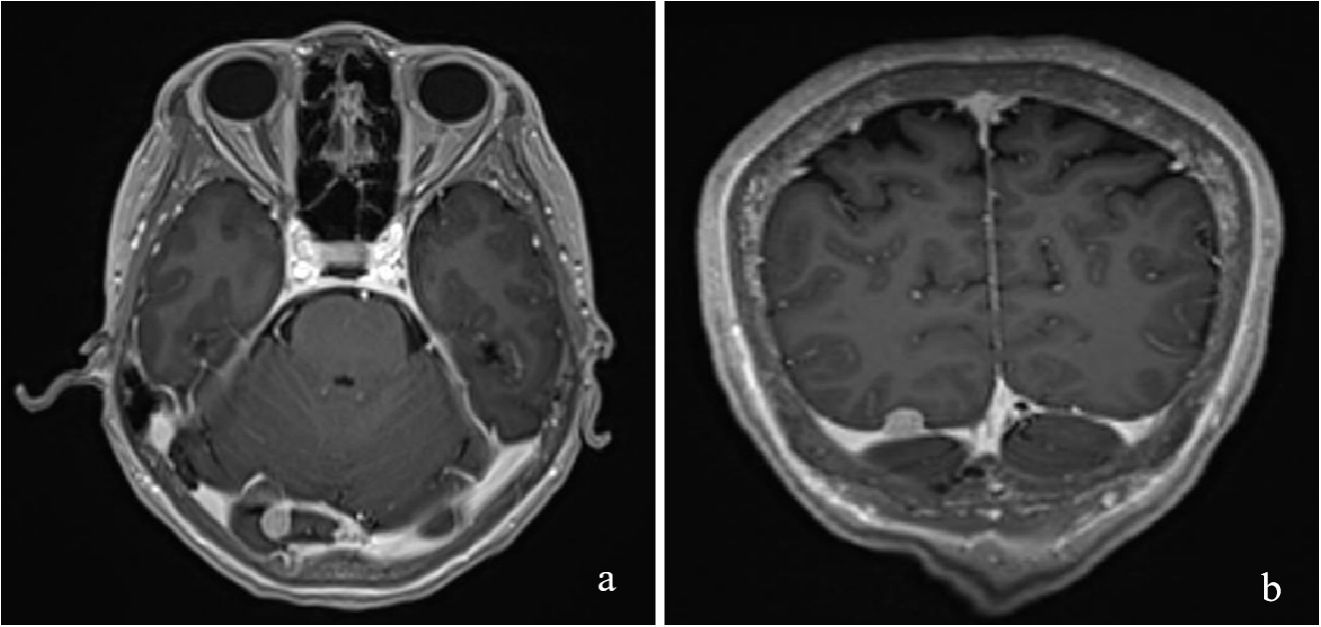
Lesions shown on the head MRI:coronal view (a) and axial view (b).
